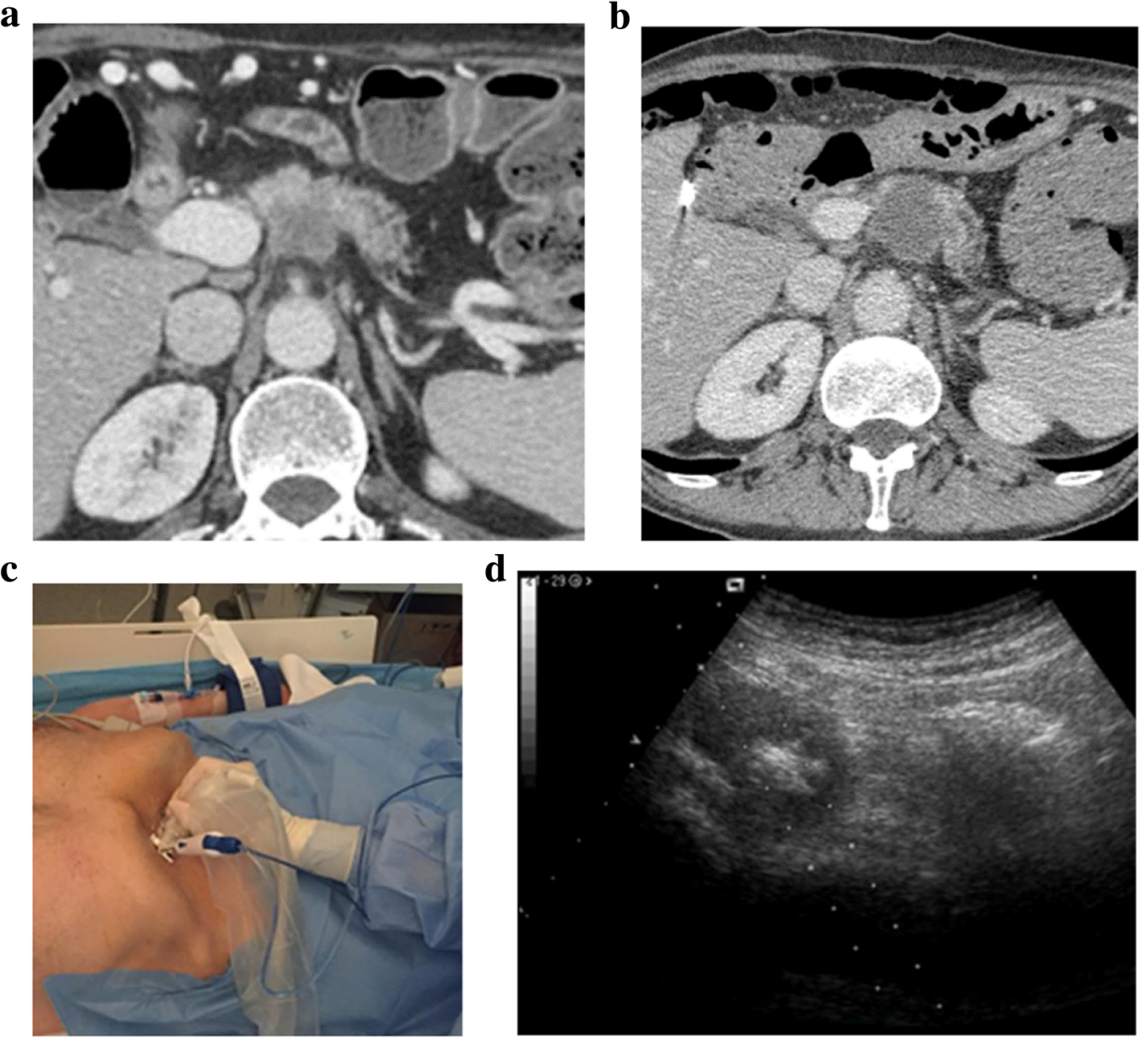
Computed tomography of an unresectable pancreatic ductal adenocarcinoma before (a) and after radiofrequency ablation (b). Patient presented with a locally advanced pancreatic ductal adenocarcinoma (40 × 35 mm) involving the celiac trunk. After twelve cycles of FOLFIRINOX chemotherapy, RFA of the lesion was performed. After the procedure (b), a homogeneous well-demarcated hypodense necrotic area confirmed the success of the procedure. No complications were reported. c Radiofrequency ablation of a ductal adenocarcinoma (patient setting). The procedure is performed in absolute sterility, in a surgery room with anaesthesia support. The ablation needle is mounted on a specific support for the probe. The procedure is performed by a single skilled operator. d Radiofrequency ablation of a ductal adenocarcinoma under ultrasound guidance. Gas bubbles generated during the procedure spreads centrifugally from the tip of the needle, permitting to monitor the margins of the ablated area in relation to the tumour borders
